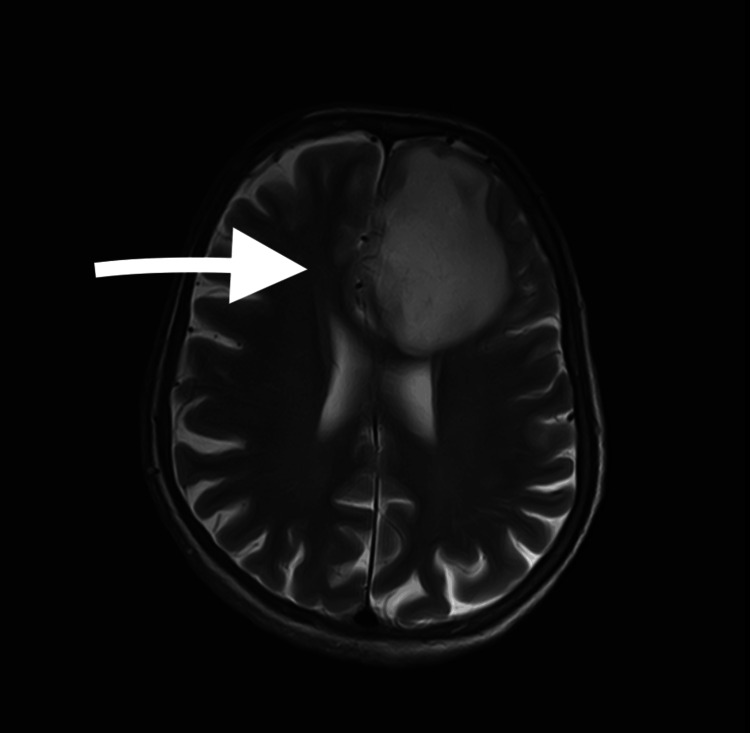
MRI image demonstrating the tumor The arrow highlights a hemorrhagic metastatic deposit in the left frontal lobe, accompanied by surrounding vasogenic edema that is causing a mass effect and a midline shift.
